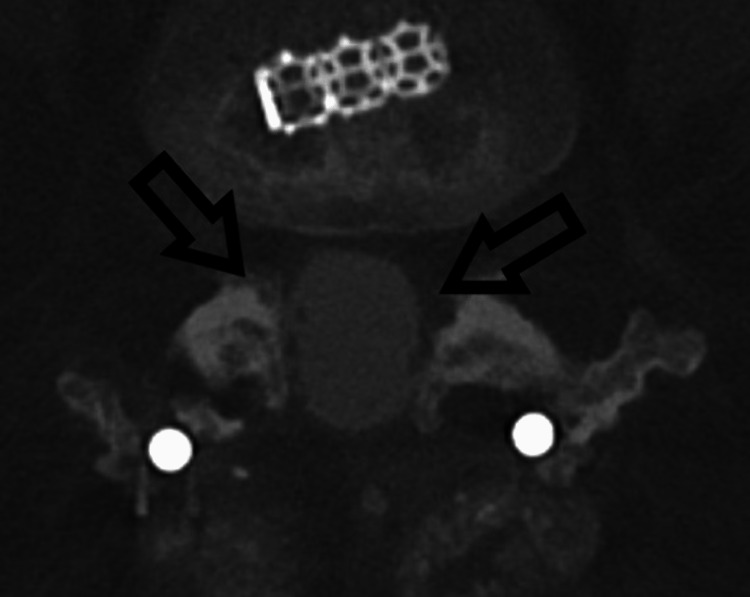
Computed tomography lumbar myelogram also demonstrates apparent adhesion of the lumbar nerve roots to the peripheral aspect of the dura (arrows).
